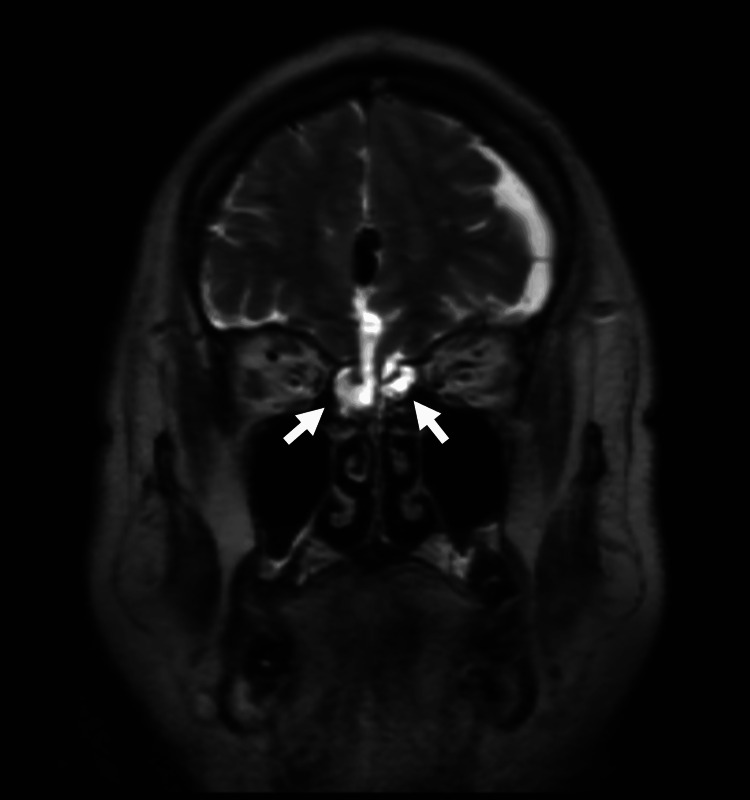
Magnetic resonance imaging (MRI) on admission, demonstrating prolapse of brain parenchymal tissue from the anterior skull base into the ethmoid sinus. The white arrows indicate bone defects at the bilateral skull base and herniation of brain tissue.
